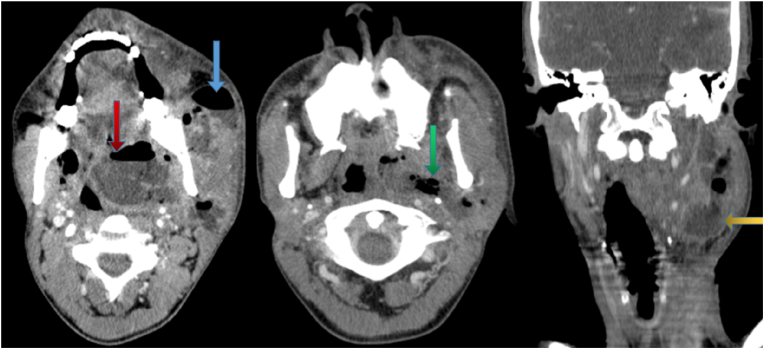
Axial and coronal contrast-enhanced neck Computed tomography reveals soft tissue abscess formation in the peritonsillar, anterior mandibular ramus, and anterior neck region.
